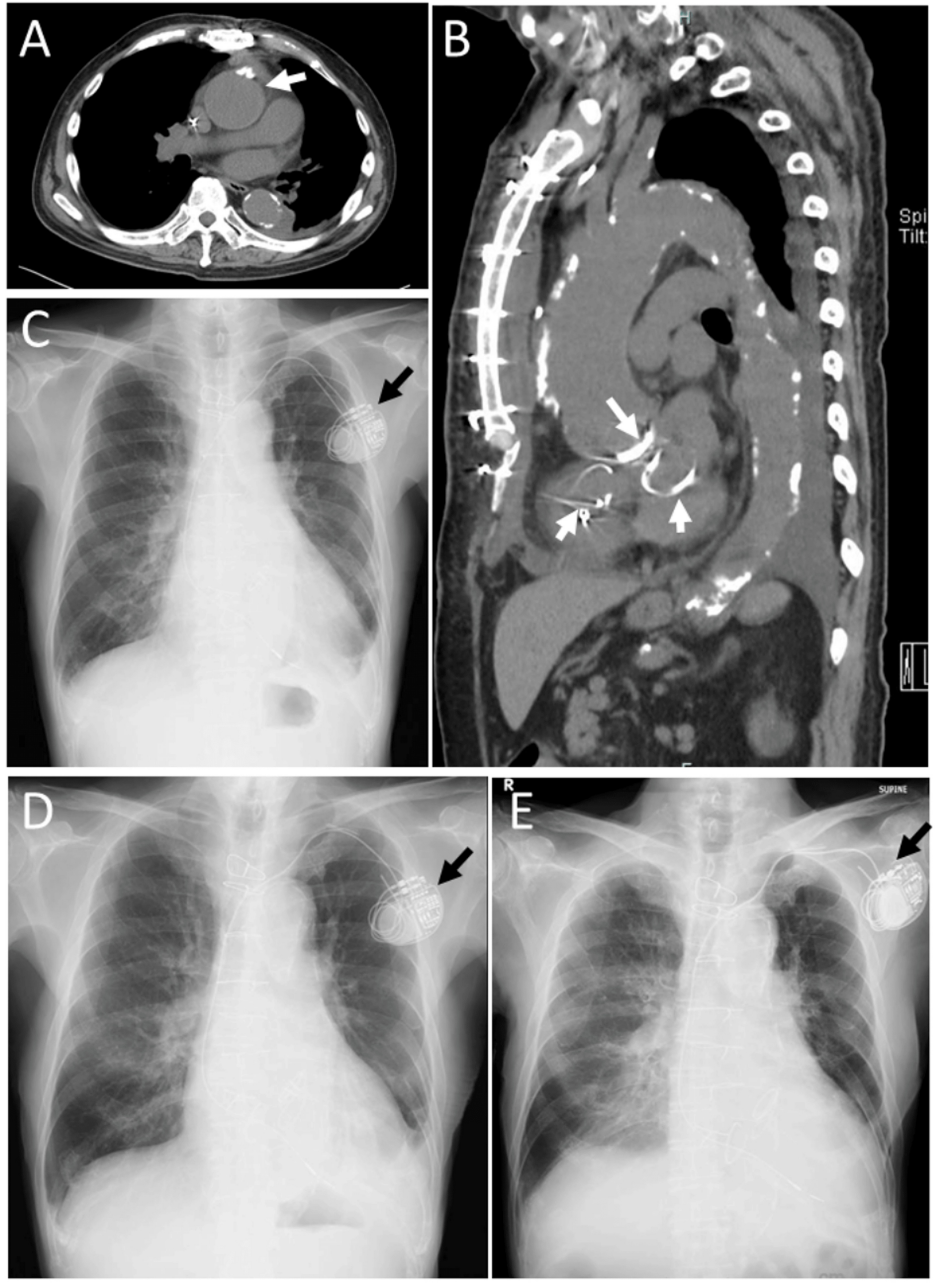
CT scans at age 73 years and plain chest X-rays at ages 73, 80, and 83 years Axial (A) and sagittal (B) CT scans obtained two weeks after cardiac surgery at age 73 years show a fusiform ascending aortic aneurysm with a diameter of 50 mm (arrow, A) and left-sided pleural effusion (A), as well as the aortic valve replacement (arrow, B) and mitral and tricuspid valve annuloplasty (short arrows, B). Plain chest X-ray films obtained one month after surgery (C), at age 80 years (D), and at age 83 years (E), one week before death, demonstrate left-sided pleural effusion in C and D. Marked cardiomegaly with bilateral pulmonary edema in the supine position is noted in E. Black arrows indicate the heart pacemaker.
